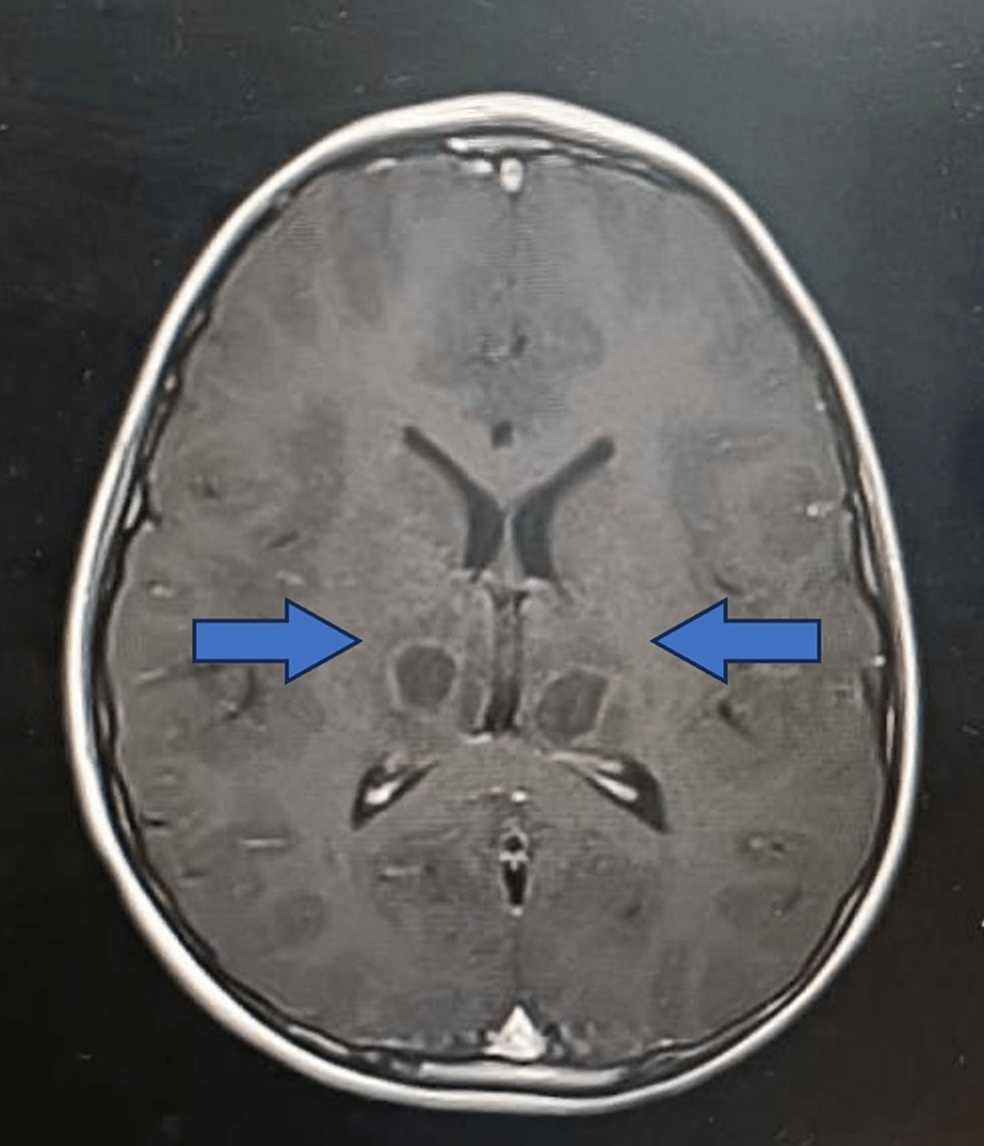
Axial plane MRI shows swelling and symmetrical abnormal signal intensities involving bilateral thalami (arrows).
